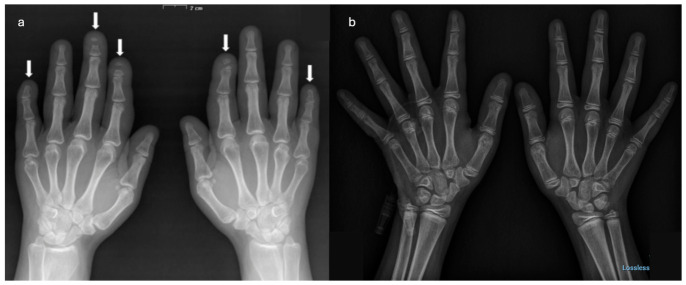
Comparative hand radiographs of the mother (a) and son (b). Anteroposterior views show advanced distal phalangeal resorption in the mother as highlighted by the arrows (a) versus absent acro-osteolysis and preserved metacarpal and joint integrity in the son (b).
